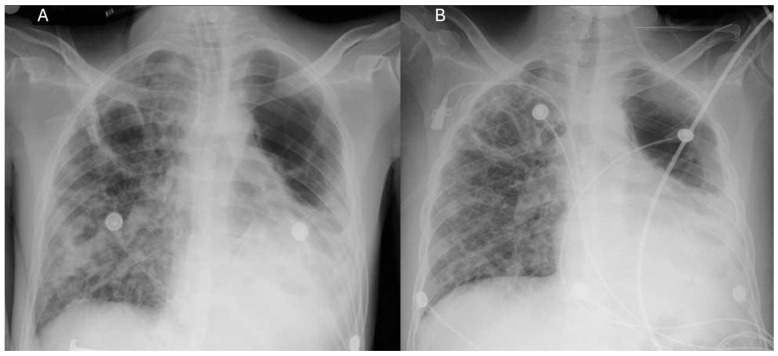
Sequential chest radiographic follow-up. (A) Endotracheal intubation: Multiple bullae in both lung fields, alveolar infiltrates in the right lung field, and bilateral perihilar atelectatic bands. (B) Post endotracheal intubation: The clouding of the left lung base persists, as do the multiple bullae in the right apex.
